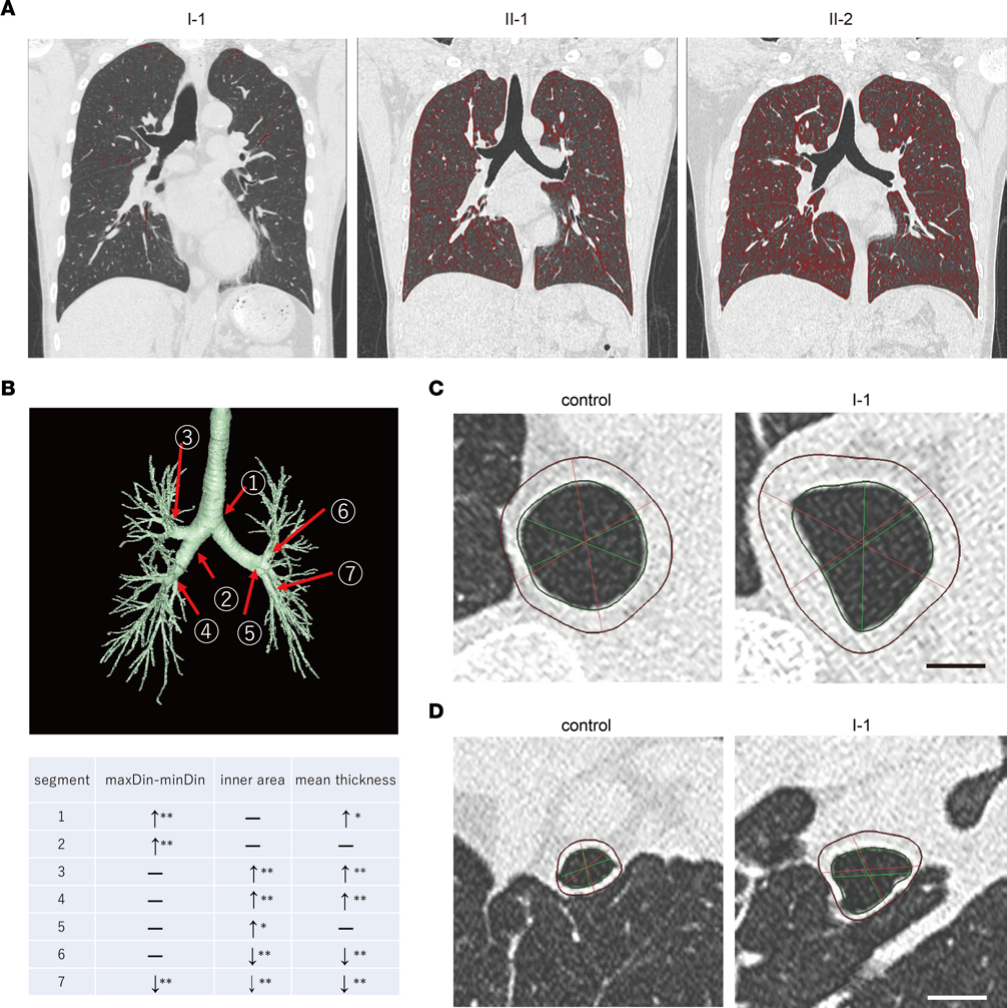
Extrarenal phenotypes of patients. (A) Emphysematous changes in patients. Low-attenuation areas (LAAs) were calculated under the threshold of –950 Hounsfield Units and are indicated in red in each chest CT image. Patients II-1 and II-2 had slight and moderate emphysematous changes in their lungs, respectively. Patient I-1 had no emphysematous change. (B) Detailed 3D analyses of bronchial tubes using chest CT. 3D bronchial tree of patient I-1 with indicated measured segments and analysis results. maxDin-minDin: difference between maximum inner diameter and minimum inner diameter at each segment. P* < 0.05; *P* < 0.01; Mann-Whitney *U* test. –, not significant. *↑*, increase; ↓, decrease. (C) Cross-sectional images of main bronchi (segment 1) of patient I-1 and an age- and sex-matched healthy control volunteer showing a deformed and thickened bronchus in patient I-1. Scale bar: 1 cm. (D) Cross-sectional images of bronchial tubes (segment 3) of the healthy control volunteer and patient I-1 showing lost bronchial tubes, as well as thickened bronchial tubes, in patient I-1. Scale bar: 1 cm.
